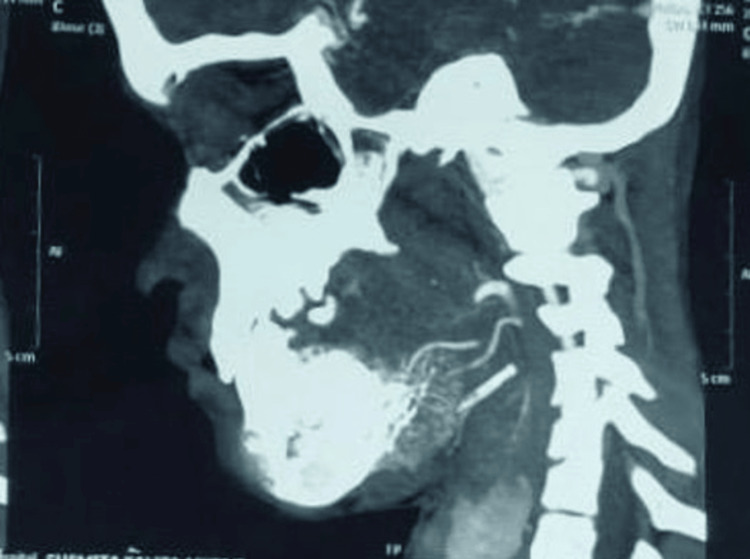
Sagittal CT angiogram showing the facial artery as a feeder vessel. Slice thickness: 1 mm at 1 mm interval.
